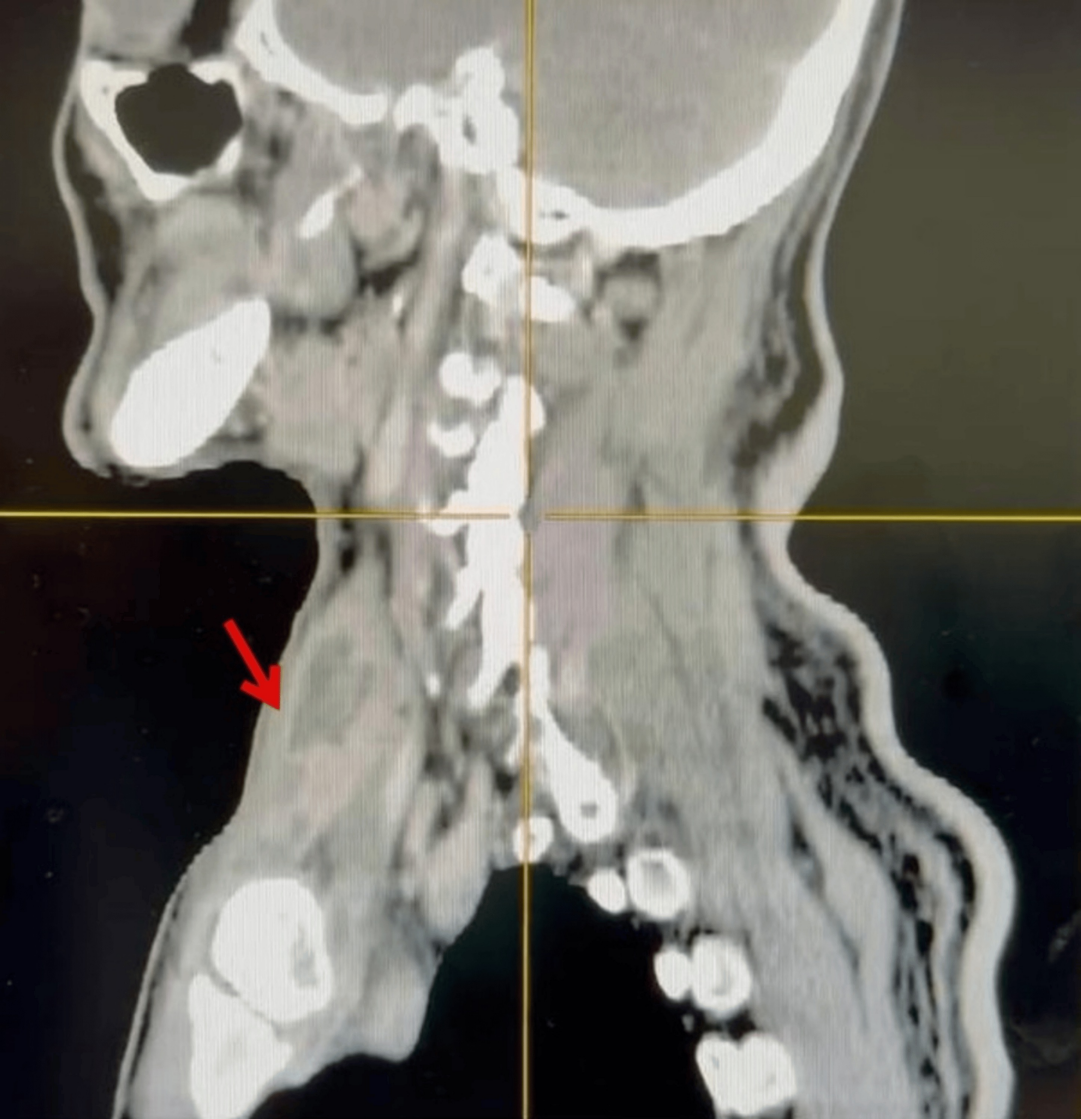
Sagittal view of CECT neck showing collection within the inferior right SCM muscle CECT: contrast-enhanced computed tomography; SCM: sternocleidomastoid
